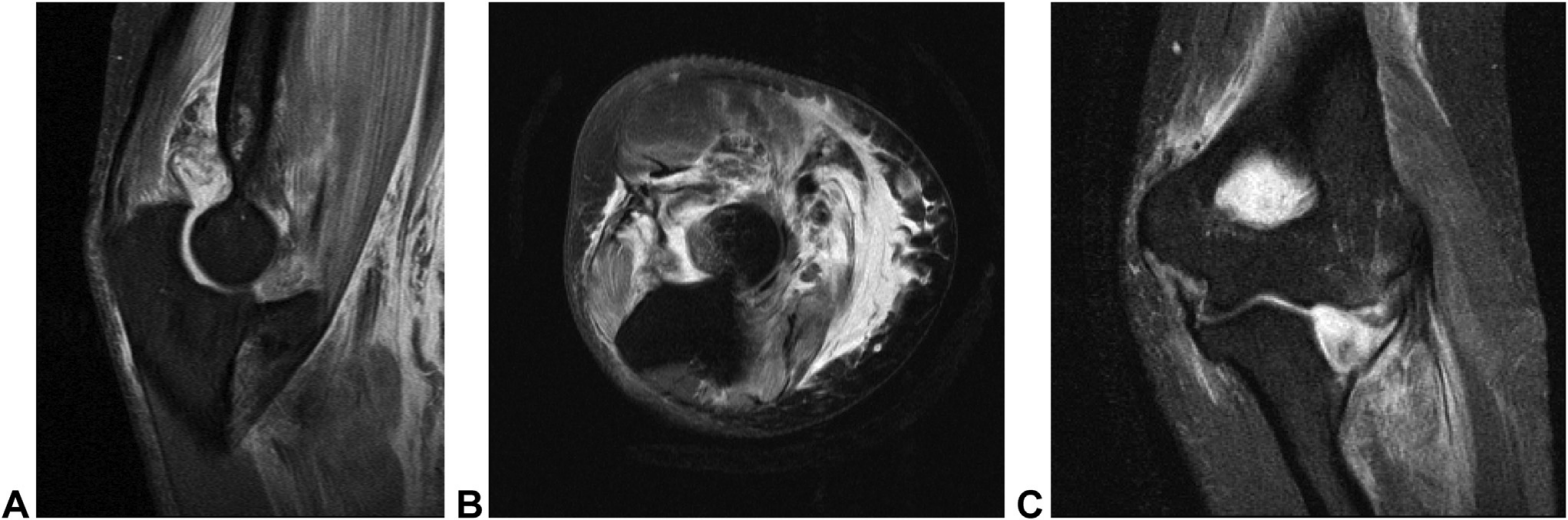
A Sagittal, B axial, and C coronal elbow MRI at presentation demonstrate anteromedial dislocation with posterior radial head impaction fracture.
